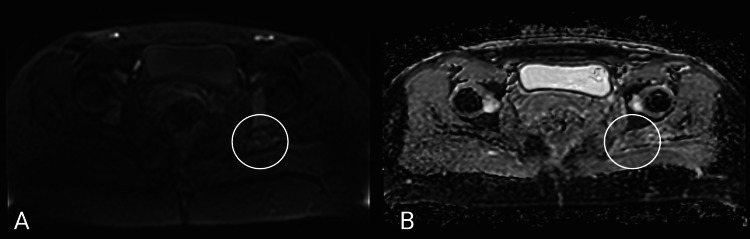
Diffusion imaging of the left sciatic nerve lesion. (A) Diffusion-weighted imaging (DWI) shows the lesion (white circle) without significant diffusion restriction. (B) The corresponding apparent diffusion coefficient (ADC) map confirms the absence of restricted diffusion.
